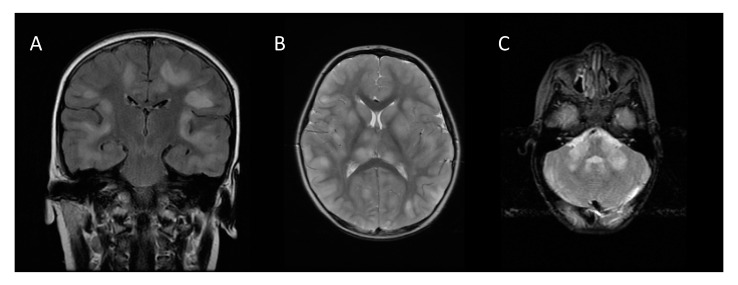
Typical magnetic resonance imaging features of ADEM: (A) coronal fluid-attenuated inversion recovery (FLAIR) image showing asymmetrical bilateral subcortical white matter abnormalities; (B) axial T2-weighted image depicting asymmetrical bilateral white and gray matter abnormalities; (C) axial T2-weighted image showing typical cerebellar peduncle lesions.
